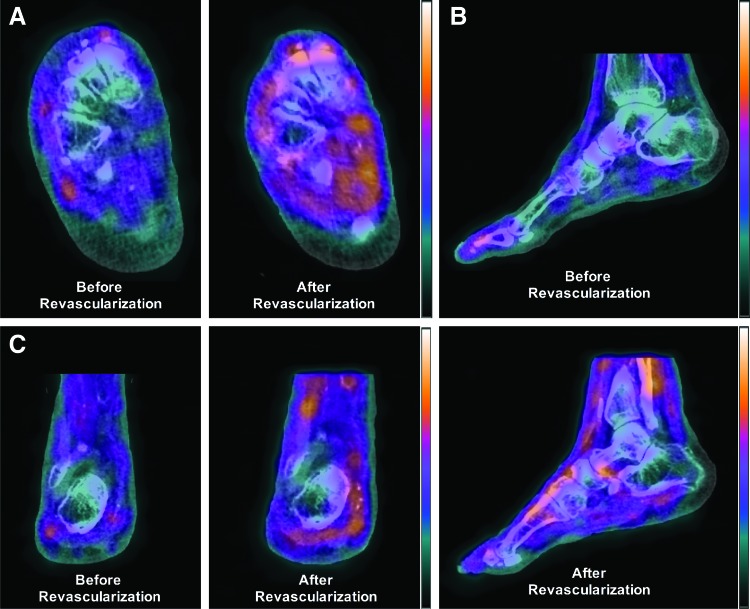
SPECT/CT perfusion imaging in a CLI patient before and after revascularization. Coregistered and fused SPECT/CT images demonstrate increased radiotracer uptake and improved microvascular perfusion in the foot in the (A) axial, (B) sagittal, and (C) coronal views following balloon angioplasty of the SFA and popliteal artery. SPECT, single-photon emission computed tomography. Color images are available online.
